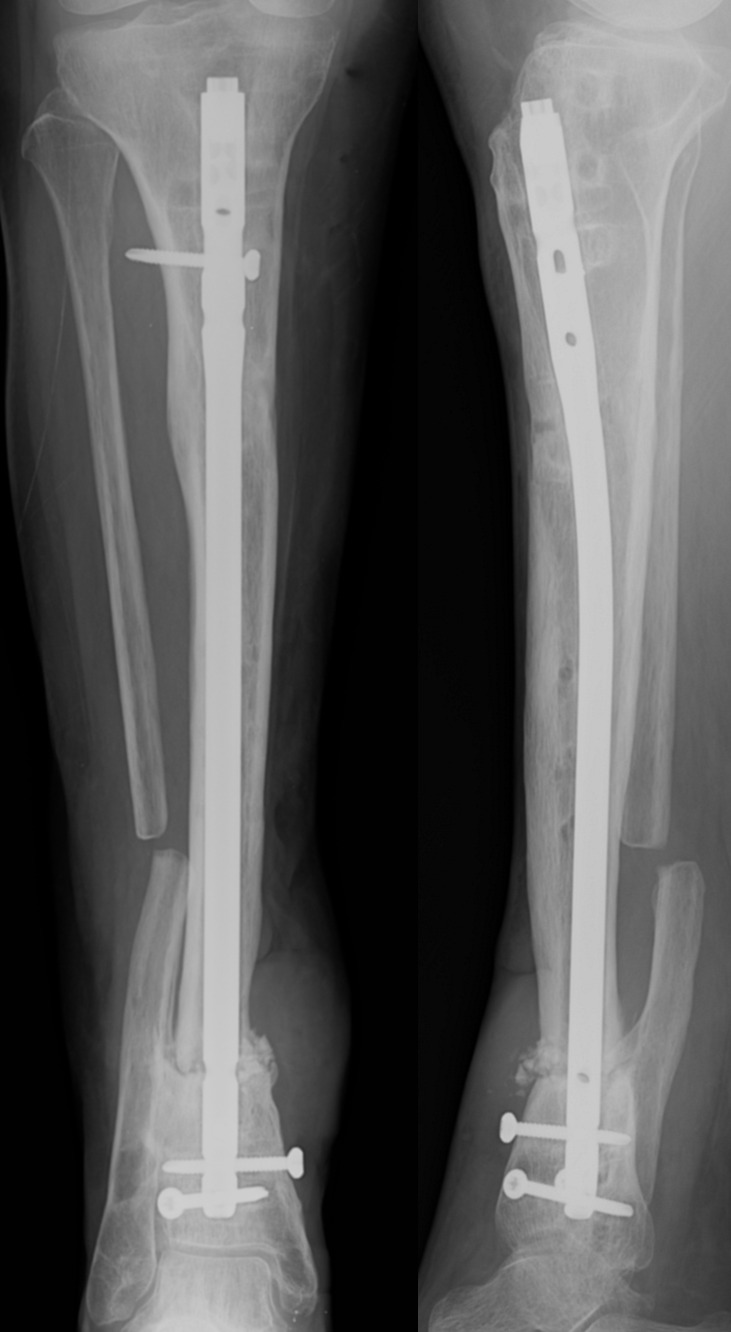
Follow-up X-ray after nail dynamization, in CFRI case report.
